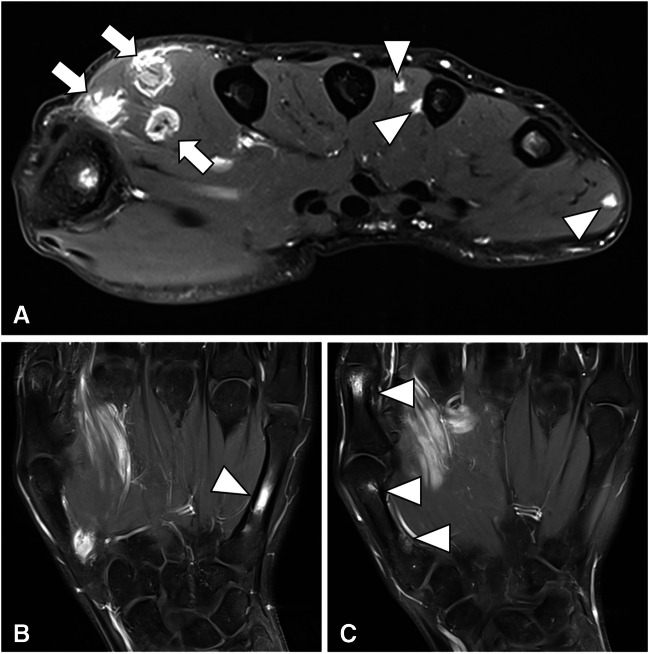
Contrast-enhanced T1-weighted MRI of the hand reveals several enhancing small (A, arrowheads) and medium-sized (A, arrows) nodular lesions of the dorsal hand muscles. Further, it depicts enhancing bone lesions in the first and fifth metacarpals (C, arrowhead). Simultaneous presence of bone and soft tissue lesions indicates a systemic disease, whereas the nodular shape is suggestive of a granulomatous disease
